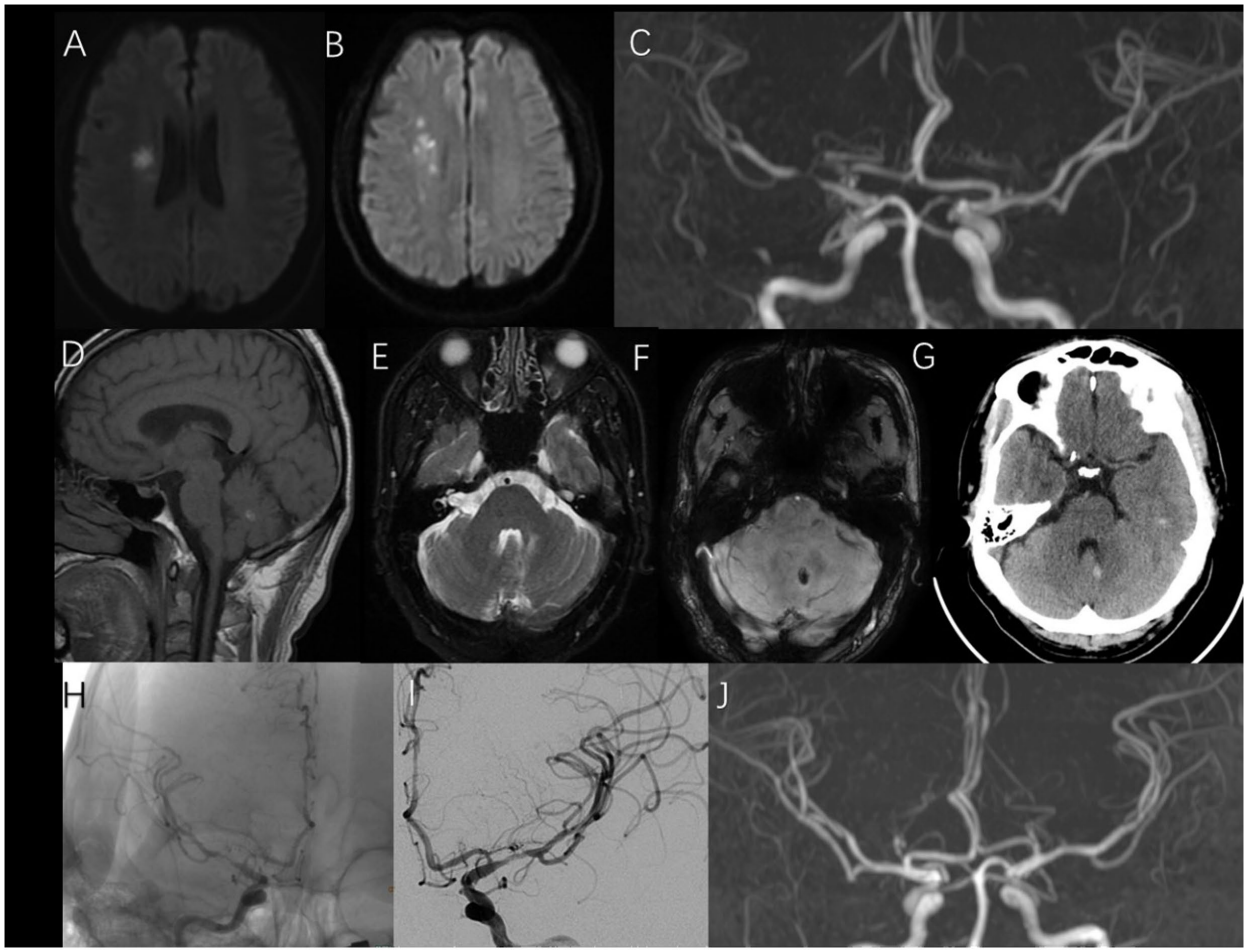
(A, B) Ischemic stroke lesion in the paraventricular region; (C) Stenosis in the right middle cerebral artery; (D–G): Cerebral cavernous malformation in the left cerebellum (D): T1, (E): T2, (F): susceptibility-weighted imaging, (G): computed tomography; (H): Stenosis in the right middle cerebral artery; (I): Successful dilation in the right middle cerebral artery; (J): Right middle cerebral artery in follow-up period.
